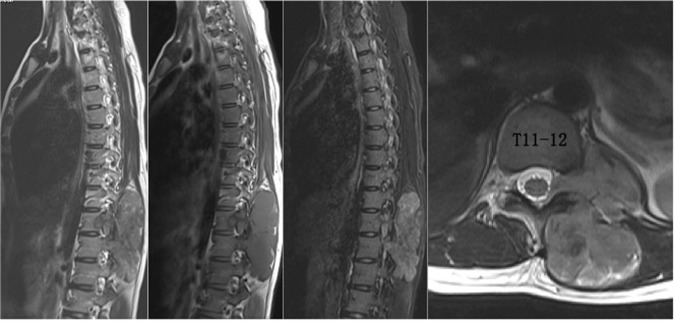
A case of a 17-year-old female with leiomyosarcoma at T11–12. The patient who complained of feeling a protruding mass in the back was found with a lesion in the thoracic spine on MRI scans. MR images show that the tumor involves the left side of T11–12 vertebra (zone 1–5, A–C, WBB).
